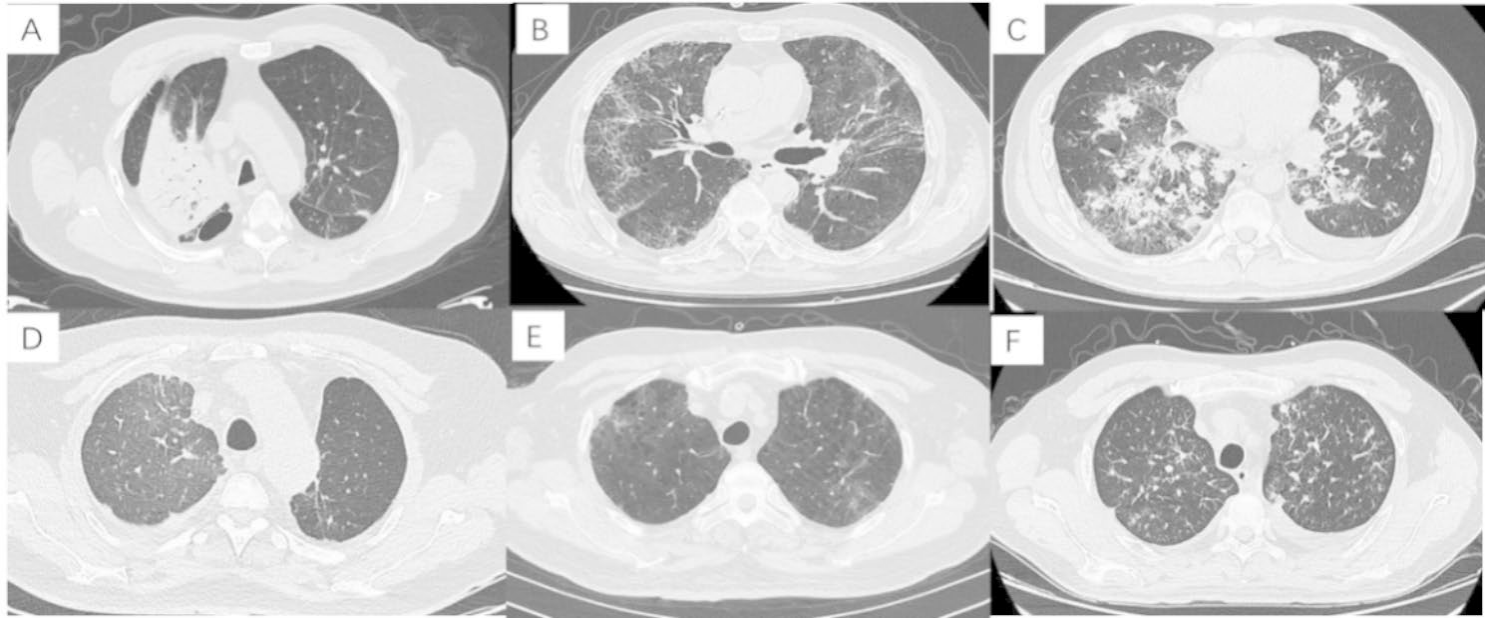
CT characteristics of CIP: A: OP pattern: extensive consolidation in right upper lobe; B: NSIP pattern: GGO and reticular shadows in both lungs; C: AIP pattern: multiple consolidation in both lungs, reticular shadows with GGO; D: HP pattern: GGO in right upper lobe; E: HP pattern: GGO with nodule shadows in both upper lobes; F: atypical changes: bronchitic changes in both upper lobes
